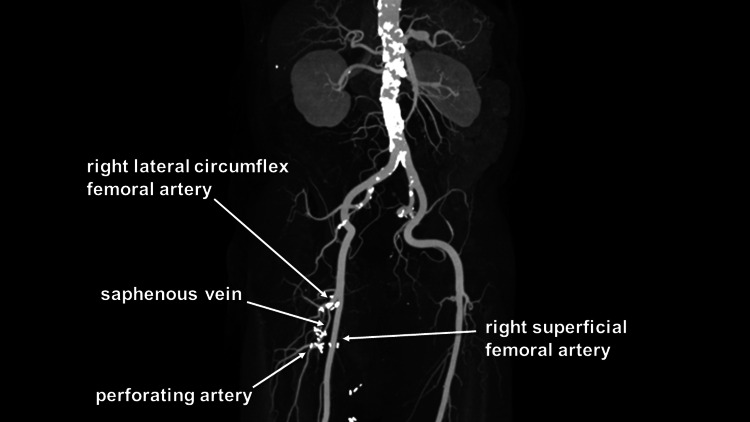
Postoperative contrast-enhanced CT Contrast-enhanced CT revealing a great saphenous vein anastomosed between the right lateral circumflex femoral artery and the perforating artery CT: computed tomography
